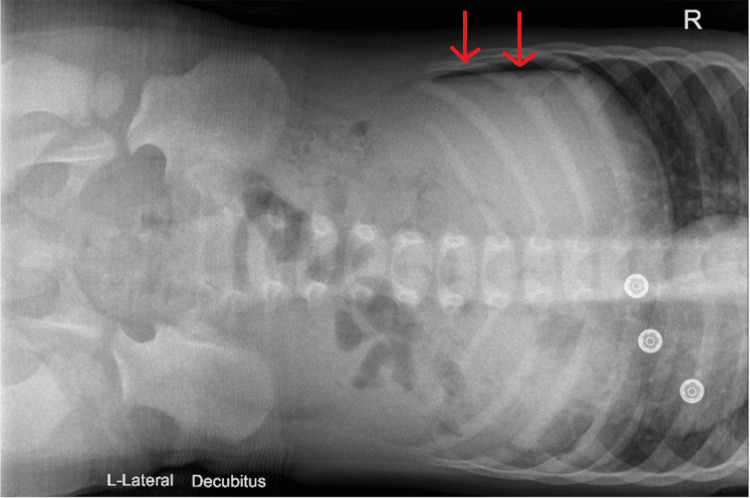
Cross-table lateral abdominal radiograph confirming free intraperitoneal air A small rim of free air is visualized (arrow), consistent with pneumoperitoneum.
